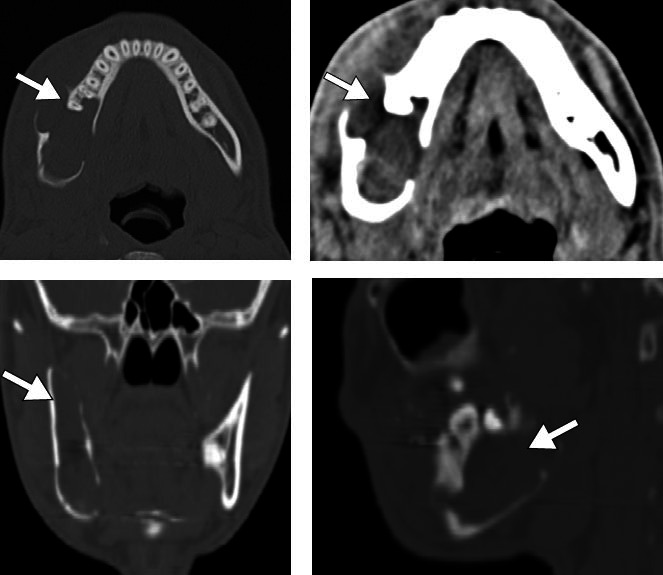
Odontogenic keratocyst in a 22-year-old male patient. CT images
